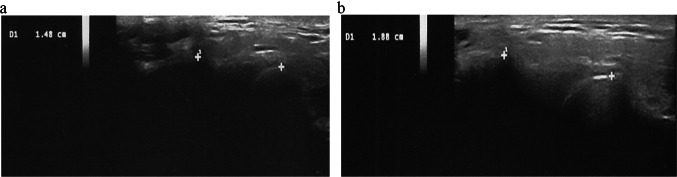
a Ultrasound image of temporomandibular joint in a SSc patient treated with protocol 2: distance condyle-head temporal bone at the point of maximum mouth opening before the treatment. The calipers are placed: in the middle point of the convexity of the condyle and on the temporal articular tubercle. b Ultrasound image of temporomandibular joint in the same SSc patient after treatment with protocol 2
